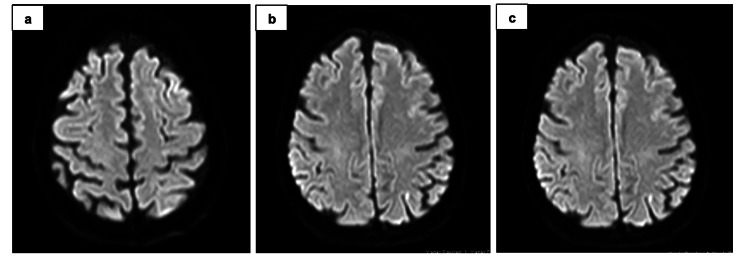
DWI obtained on Day 1 following the symptoms onset demonstrated no evidence of diffusion restriction in the brain parenchyma (a-c) DWI: Diffusion-weighted imaging
